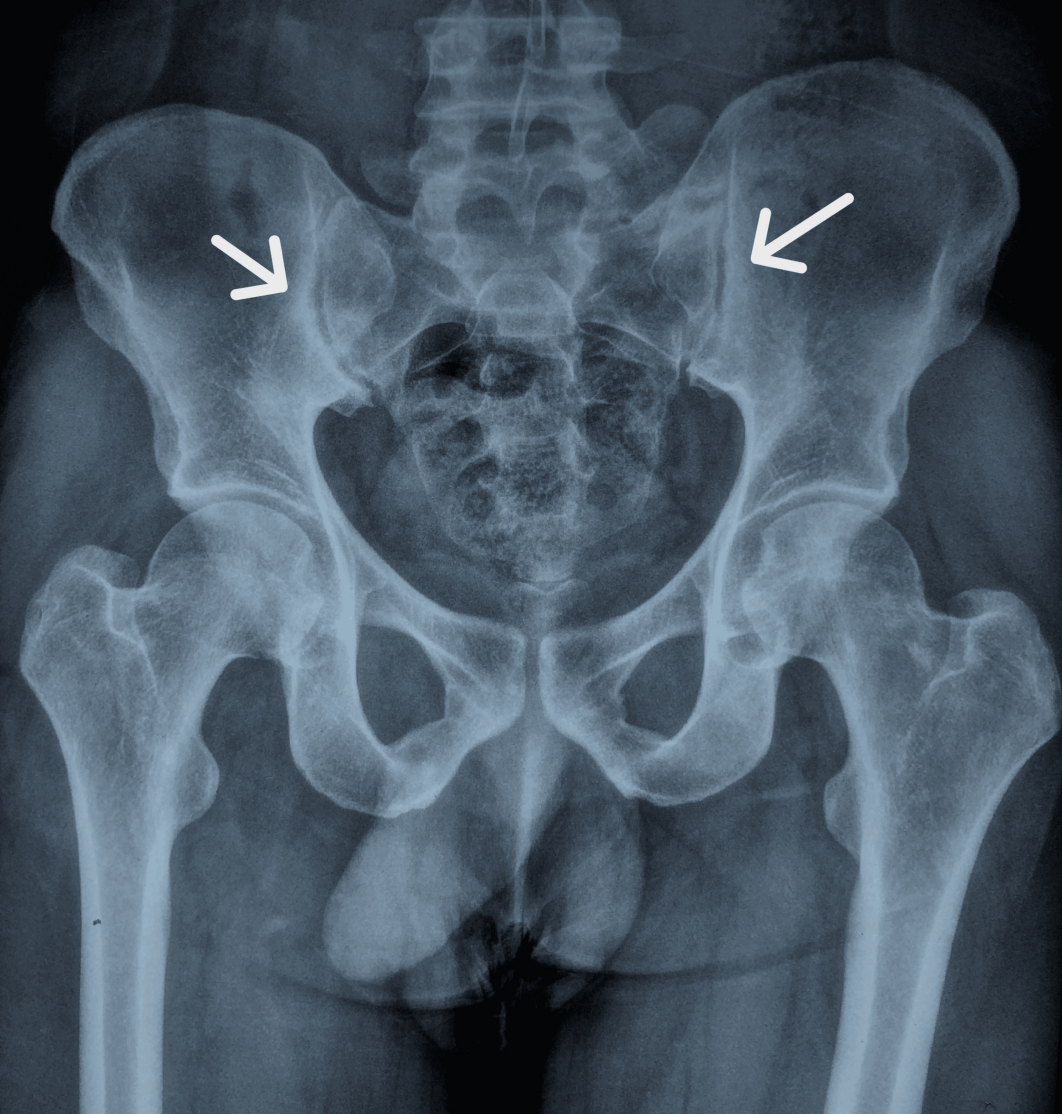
Pelvic X-ray showing subchondral sclerosis and articular surface irregularity of the sacroiliac joint.
